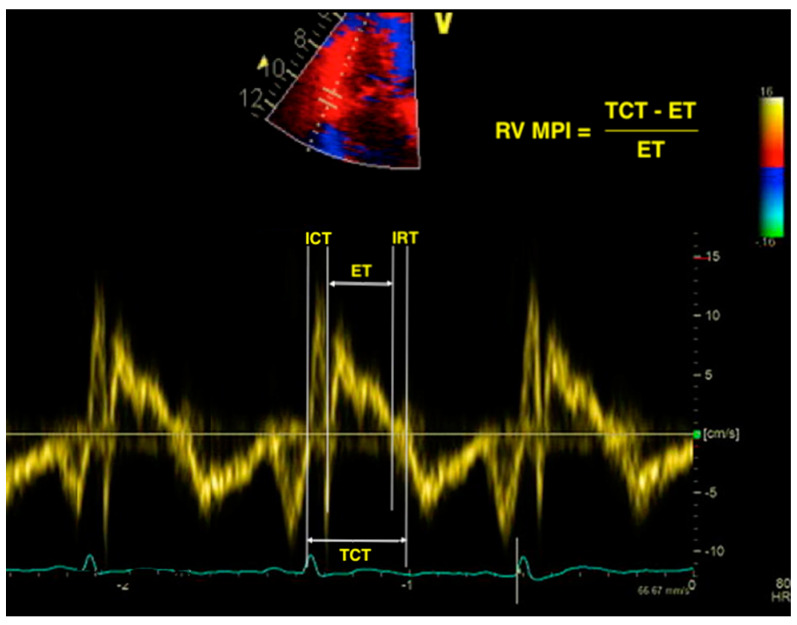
Calculation of right ventricular myocardial performance index (RV MPI) using the tissue Doppler imaging (TDI) method. RV—right ventricle; MPI—myocardial performance index; TDI—tissue Doppler imaging; ICT—isovolumic contraction time; ET—ejection time; IRT—isovolumic relaxation time; TCT—total contraction time.
